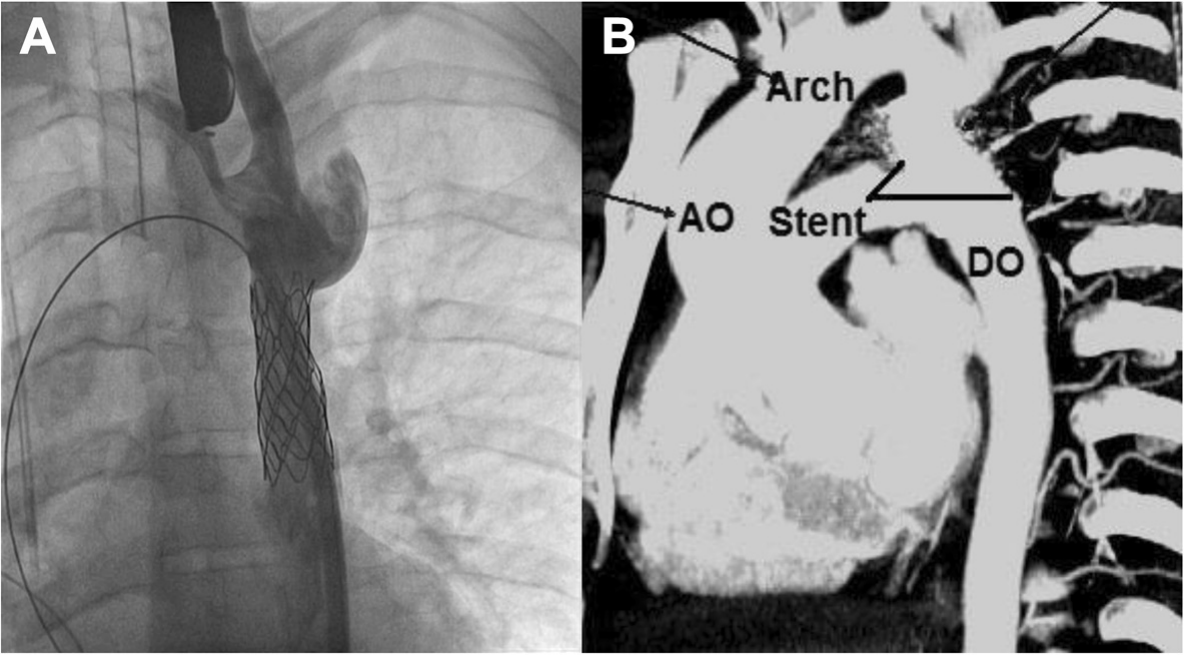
a Aortogram showing placement of a stent across the coarctation of the aorta. b Follow-up CT angiogram showing a patent stent and the lumen of the aorta. (AO- Ascending aorta, DO- descending aorta)
